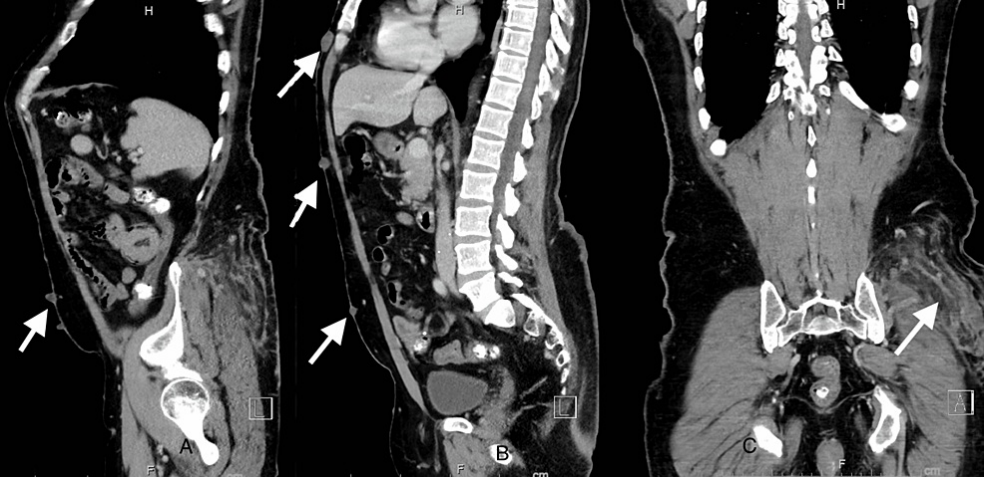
CT scan of the abdomen Arrows in A and B images show cutaneous neurofibromas. The arrow in the C image shows complex neurofibroma. CT: computed tomography
